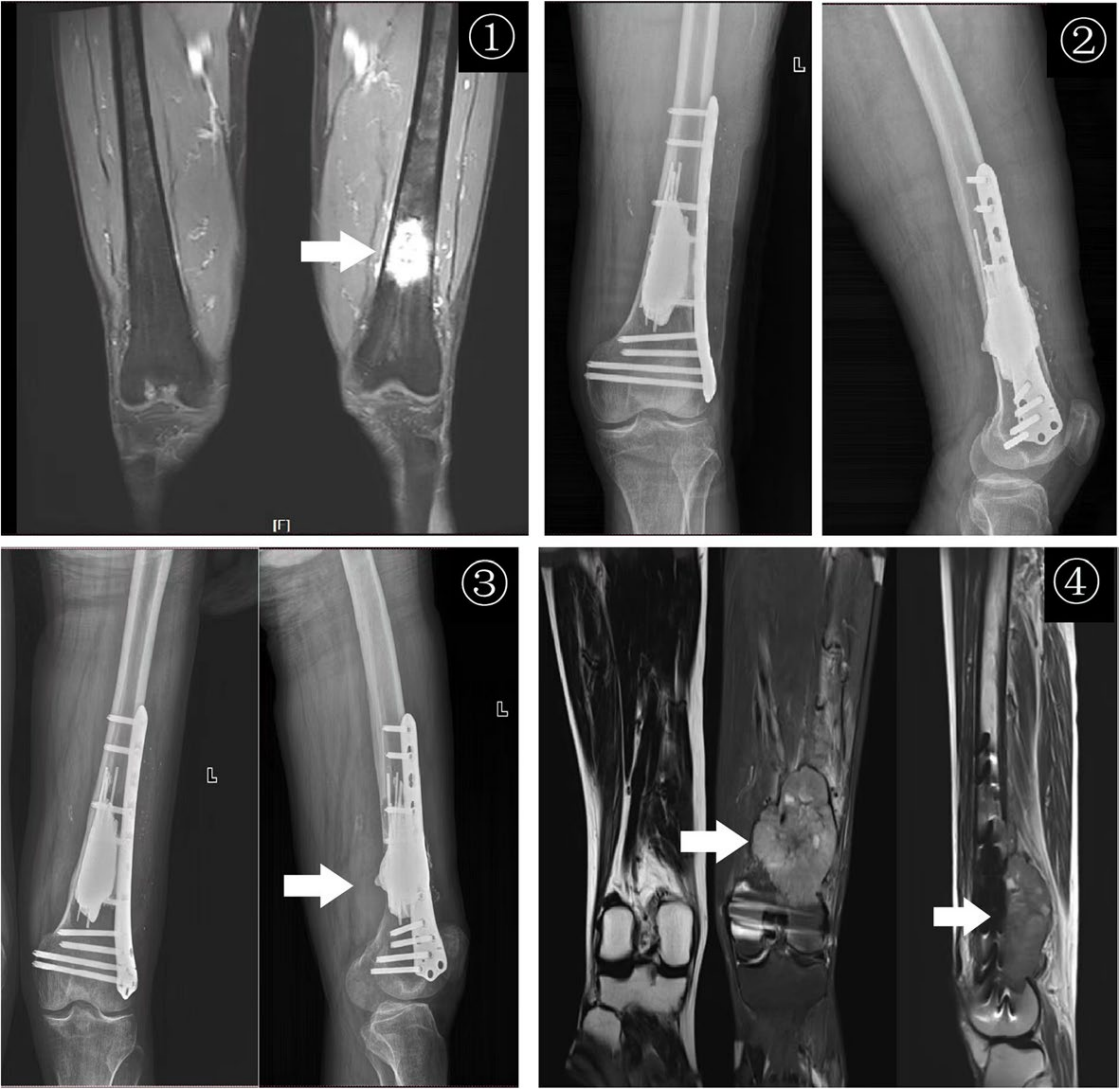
Metastatic tumor of the distal femur of renal cell carcinoma, male, 53 years old. ① Preoperative MRI T2WI (coronal) showed that the left distal femur showed a mass-like long T2 signal, indicating a tumor signal. ②–③ After 2 and 6 months of curettage and cement fixation of the tumor, the X-ray films (positive and lateral position) showed that the cement-host bone healed completely. ④ Ten months after the operation, MRI showed that the mass (coronal and sagittal) was slightly longer on T1 and T2 signal intensity, indicating tumor recurrence
